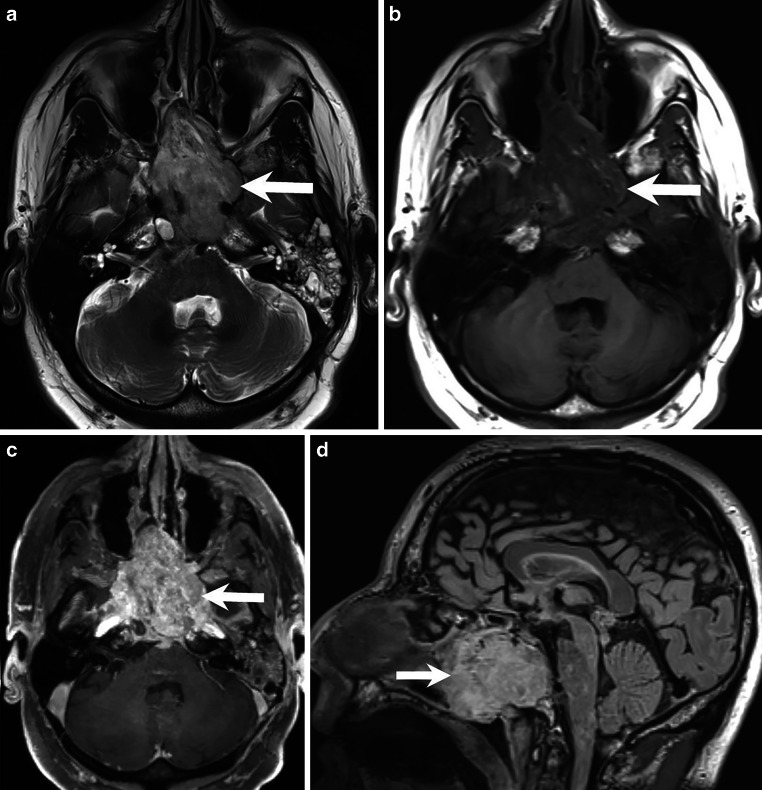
Magnetic resonance and CT images after tumor progression and increasing clinical symptoms over the past 2 years. On axial T2-weighted images, the hyperintense mass lesion infiltrated the sphenoid sinus, encircled the left internal carotid artery, and had direct contact with the basilar artery (a, *arrow*). On axial T1-weighted images, the lesion appeared hypointense (b, *arrow*). On axial T1-weighted images acquired after administration of gadolinium, the lesion appeared with marked and fairly homogeneous contrast enhancement (c, *arrow*). Sagittal fluid attenuated inversion recovery (FLAIR) images showed the size of the extended mass lesion (d, *arrow*)
